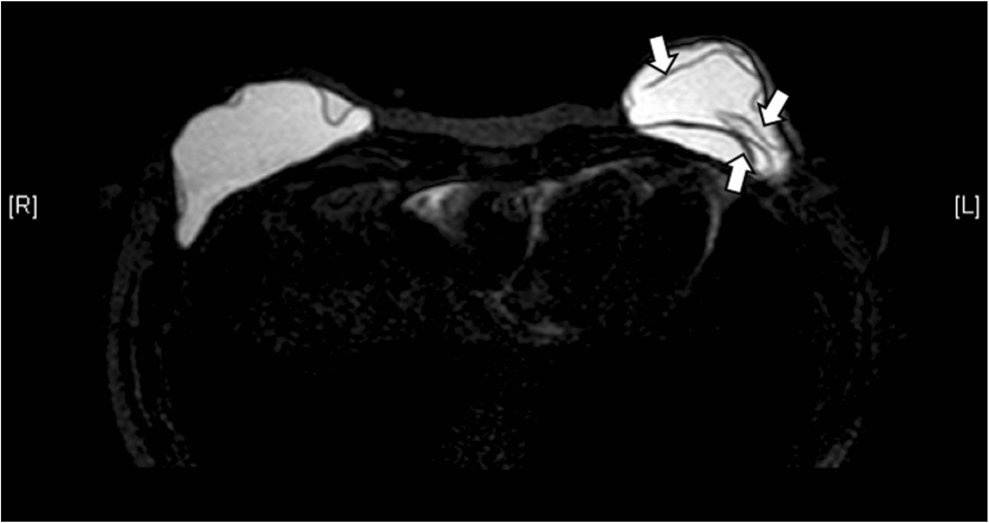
Linguine sign. Silicone-only axial MR image shows curvilinear hypointense lines (arrows) in the left breast implant, compatible with the collapsed shell floating in the high-signal silicone gel. This is called the linguine sign“Salad oil sign” or “droplet sign” occurs when there are small, hyperintense foci within the silicone gel on T2-weighted images, or hypointense foci on water-suppressed images. This is due to the presence of saline drops in silicone gel when there is an intracapsular rupture of a double lumen implant. The sign can be seen in both uncollapsed and collapsed intracapsular rupture. The presence of this sign in a single lumen silicone implant means there is water droplet in the silicone gel. Without other evidence, it is not a reliable sign of implant rupture, but its presence should prompt the search of other signs of intracapsular rupture [3].Extracapsular rupture means the leakage of the implant material beyond the fibrous capsule into the surrounding tissues. It happens when both the implant shell and the fibrous capsule are ruptured (Figs. 12 and 13). Free silicone can be seen as discrete foci, usually with identical signal intensity as the silicone gel inside the implant (Fig. 14). They appear isointense to hypointense on T1-weighted, fat-suppressed images and is of high signal intensity on water-suppressed T2-weighted images [6]. With time, silicone granuloma formation may occur, showing enhancement that may mimic breast carcinoma. Differentiation solely on the basis of imaging can be difficult. Therefore, pathological diagnosis by biopsy is warranted if there is suspicion [6]. In the case of saline-filled implant extracapsular rupture, free hydrosaline solution is usually resorbed by the body, with the remaining collapsed implant shell being the only imaging clue (Fig. 15).
